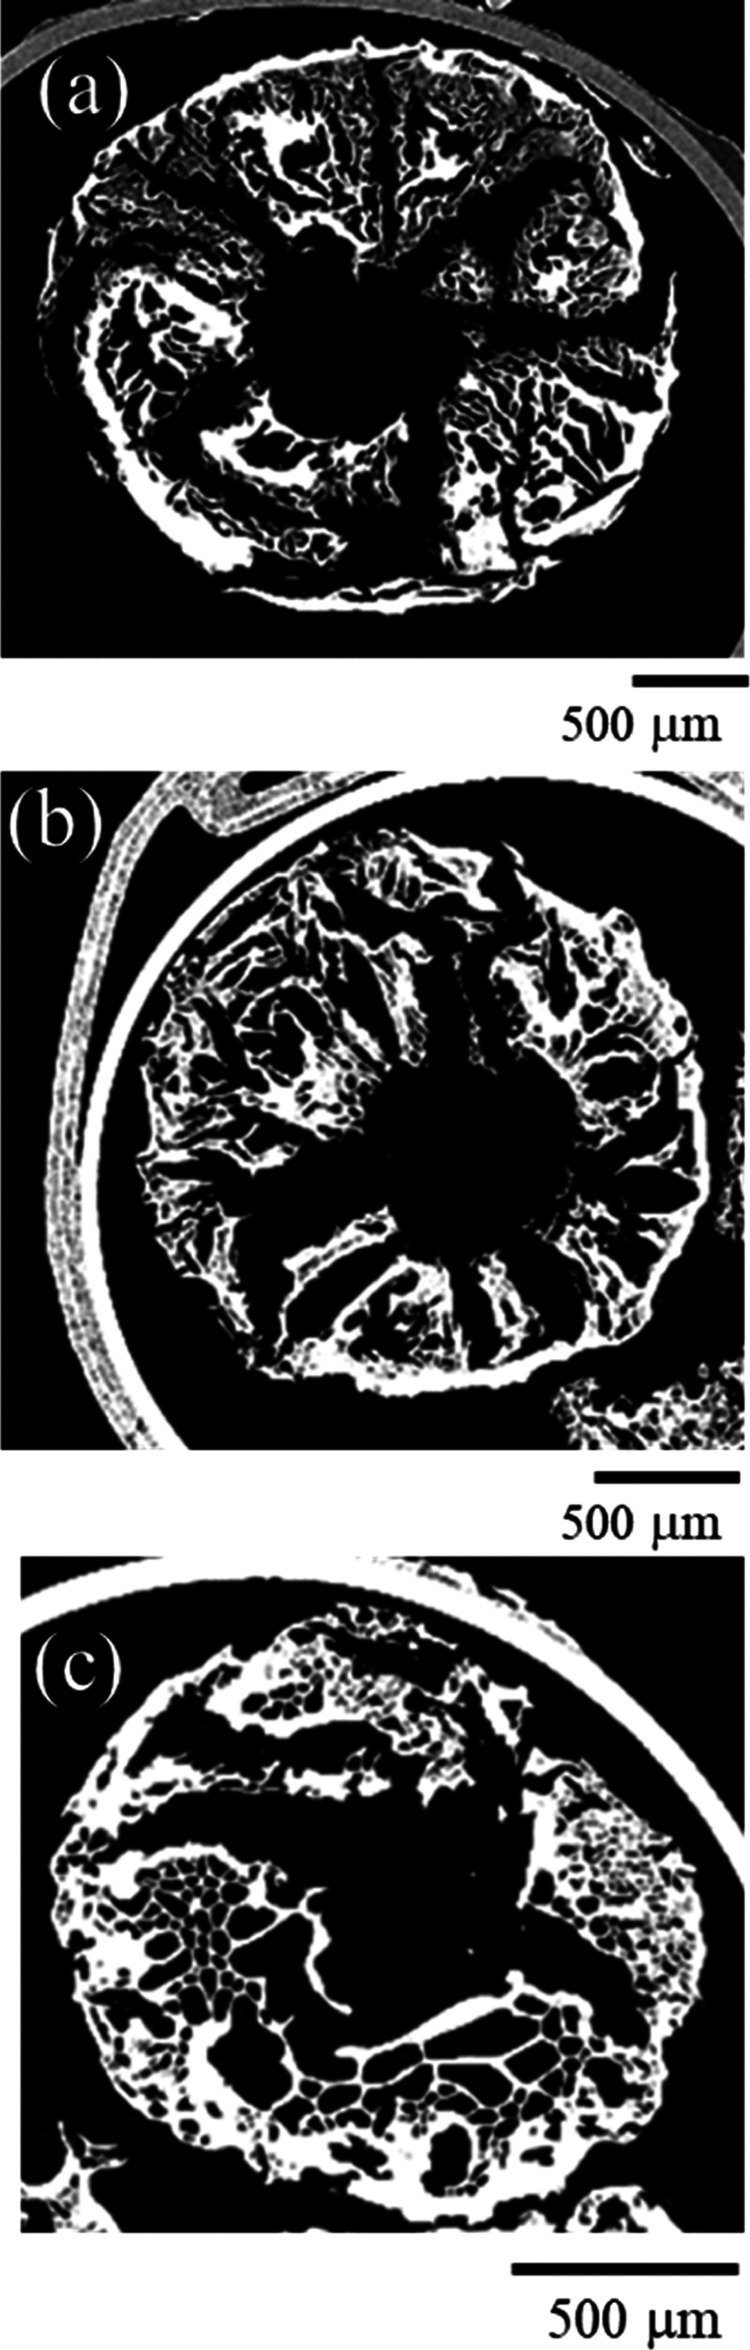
CT scan images of beads (a) 1.6 mm diameter needle, (b)
